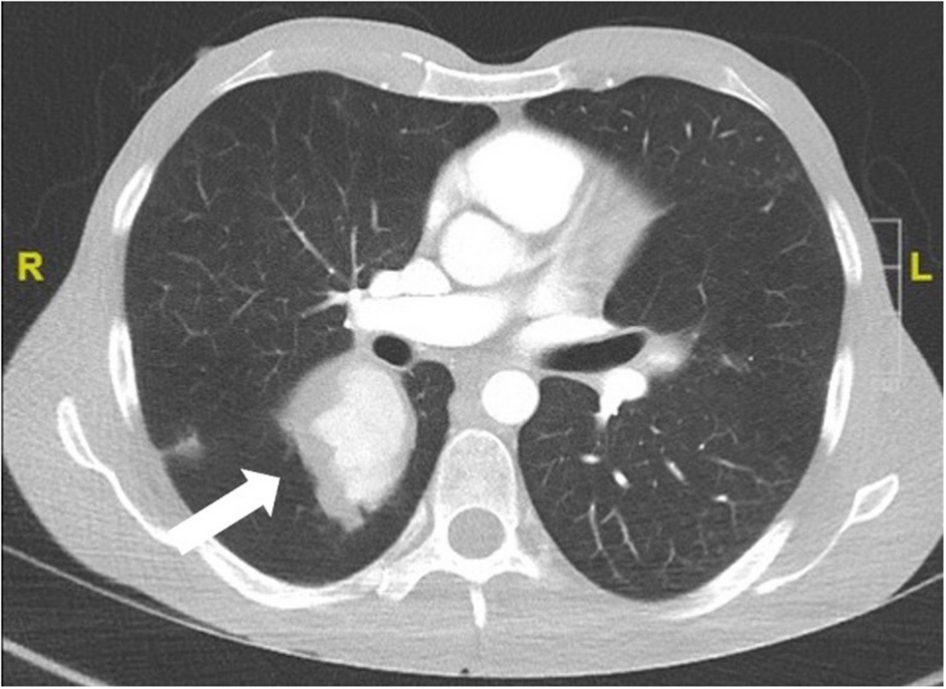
Pulmonary arterial aneurysm (CT scan with contrast agent) of a 24 year old male patient with definite BS and hemoptysis.
